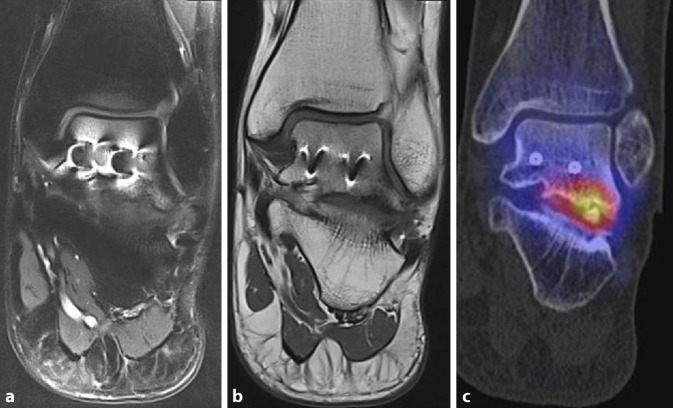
## SPECT/CT bei akzessorischen Knochen und Normvarianten
